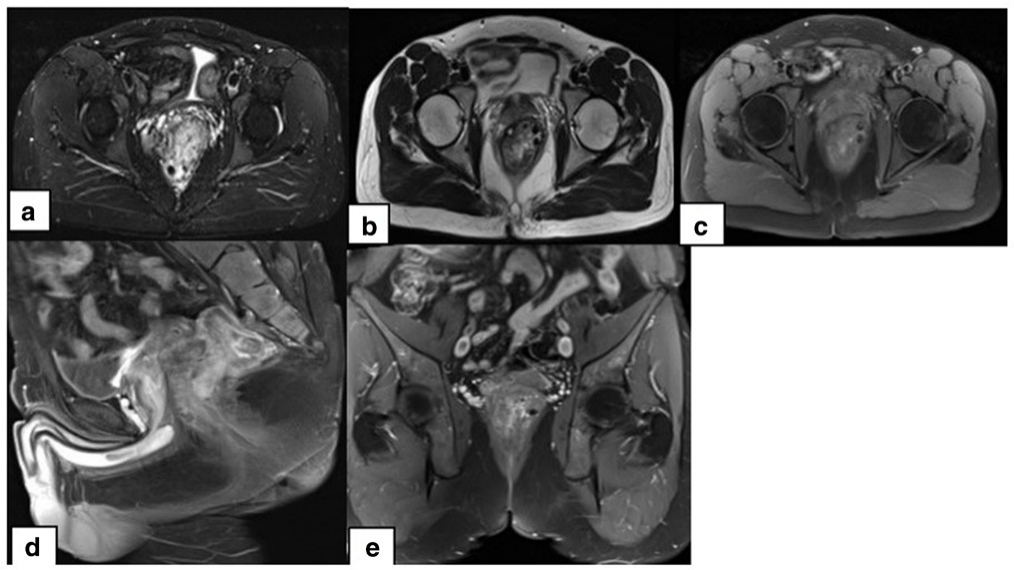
Gadolinium-enhanced MRI of the pelvis in A. Axial T1 with contrast, B. Axial T2, C. Axial STIR, D. Sagittal FATSAT with contrast and E. Coronal T1 with contrast revealing circumferential submucosal thickening of the rectum with heterogenous enhancement and multiple signal voids likely representing calcifications.
